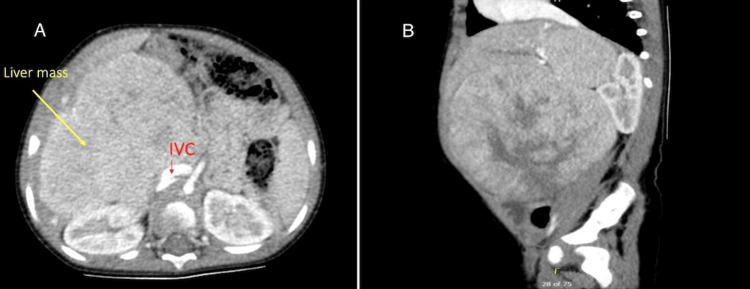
CT scan of the abdomen (axial and sagittal views)- a heterogeneous mass in the right lobe of the liver, compressing on the IVC. A: axial view; B: sagittal view
